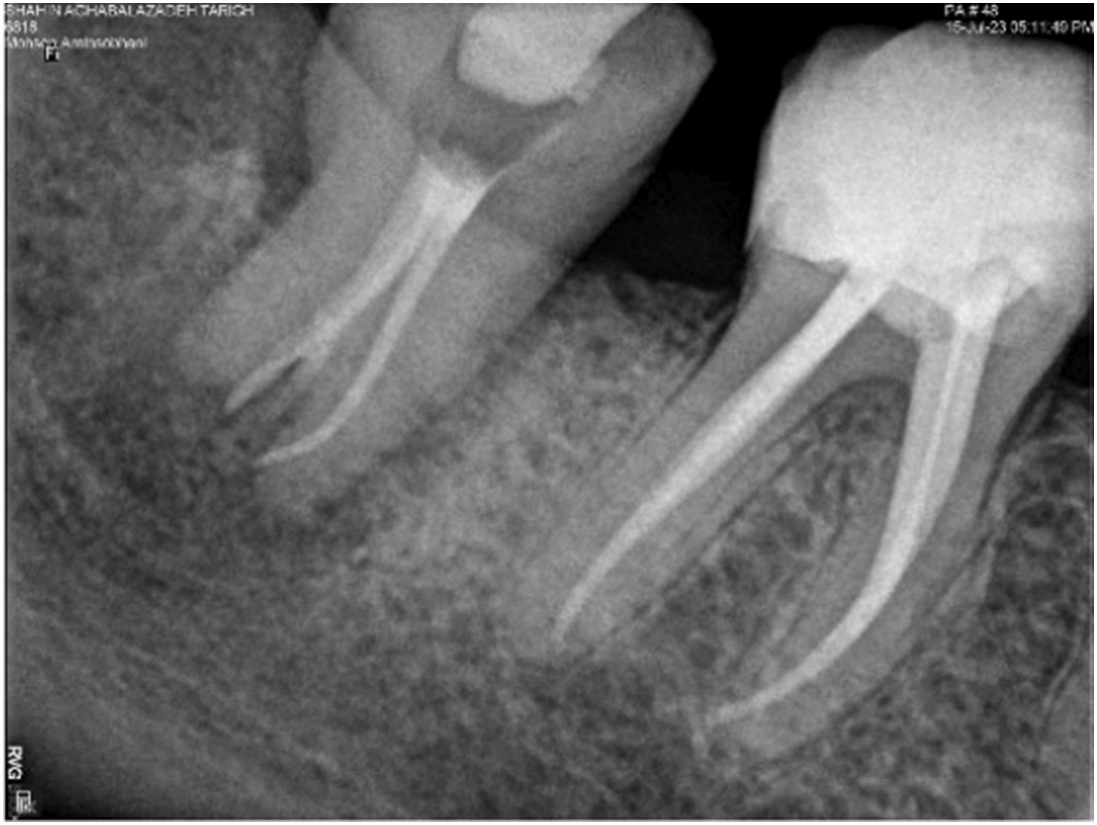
Periapical radiograph: post‐op.
